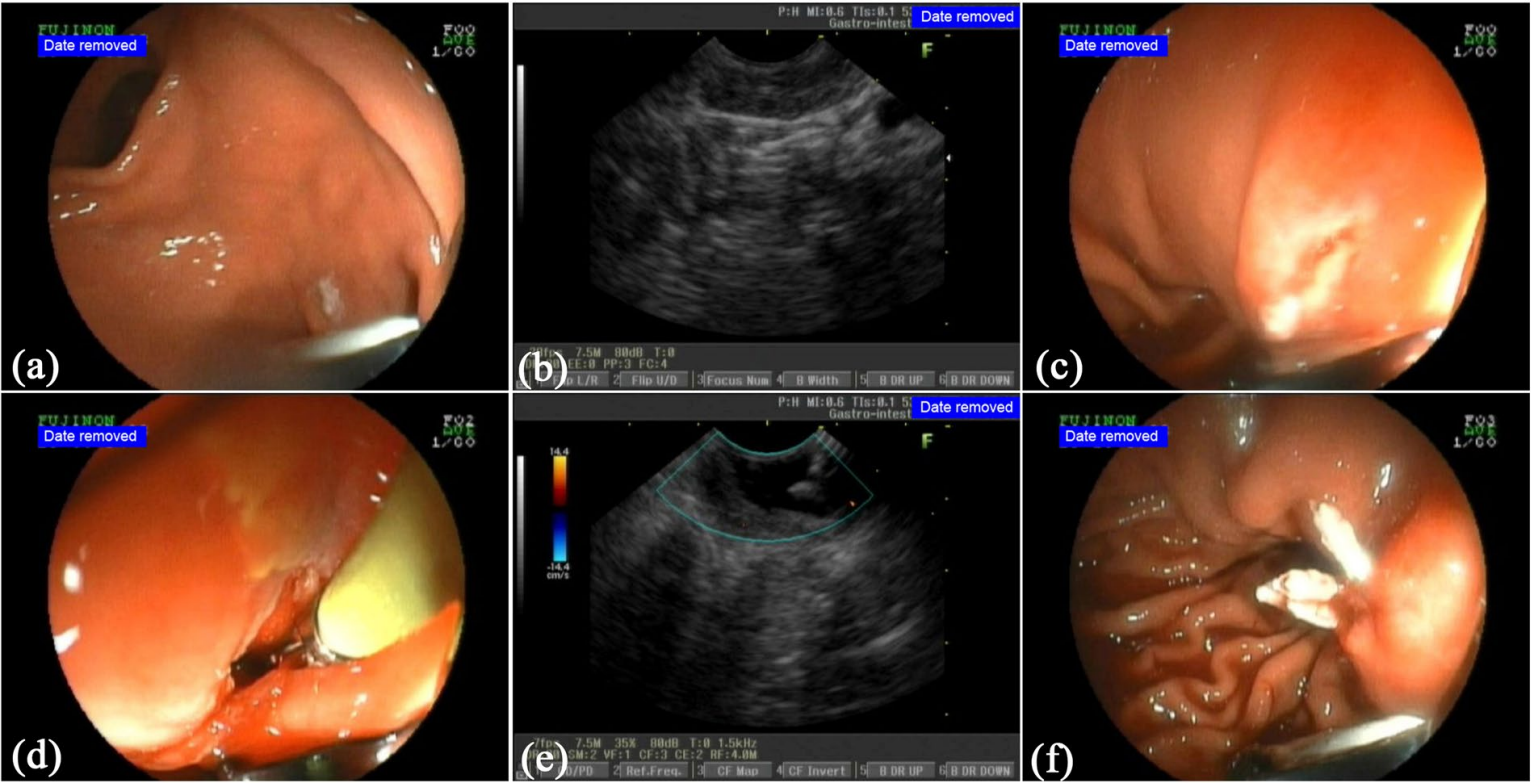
Case demonstration of the via mucosa incision EUS-guided sampling. A patient was found to have gastric wall thickening at the gastric antrum by CT. The mucosa biopsy yielded a negative result. The mucosa incision EUS-guided forceps biopsies made the diagnosis, ectopic pancreas; (a) An endoscopic view of the gastric antrum showed that the surface of the mucosa was smooth and had no obvious gastric submucosal uplift lesions; (b) An endoscopic sonography presented that the gastric wall thickening and the hyperechoic layer of submucosa was ambiguous. There was an isoechoic layer without a clear margin; (c) The mucosal layer was dissected at an appropriate depth based on EUS evaluation after submucosal administration with saline; (d) The forceps were inserted though the incision using monitoring of the endoscopic view; (e) To biopsy the submucosal lesion, the tip of the forceps were monitored by EUS; (f) After biopsy, the incision was closed with clips.
